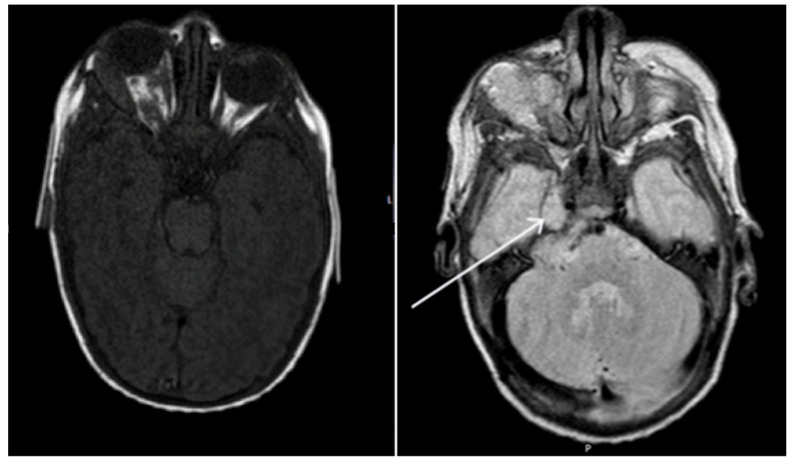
Axial T1 weighted and T2 weighted TSE brain magnetic resonance imaging of patient on presentation. Extensive right orbital lesion with marked proptosis. Extension of the lesion intracranially, with involvement of right Meckel’s cave (pointer) and the cerebellar pontine angle.
